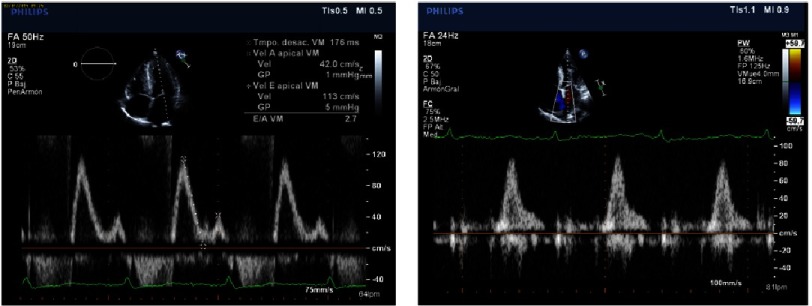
Restrictive physiology. In the image on the left, E wave high velocities measured in the mitral inflow with very low A wave velocities with abnormal deceleration time, indicates high left atrial pressure and poor compliant left ventricle. On the right hand side, the pulmonary vein Doppler velocities show absence of systolic inflow wave and high diastolic velocities, consistent with restrictive physiology as well.
